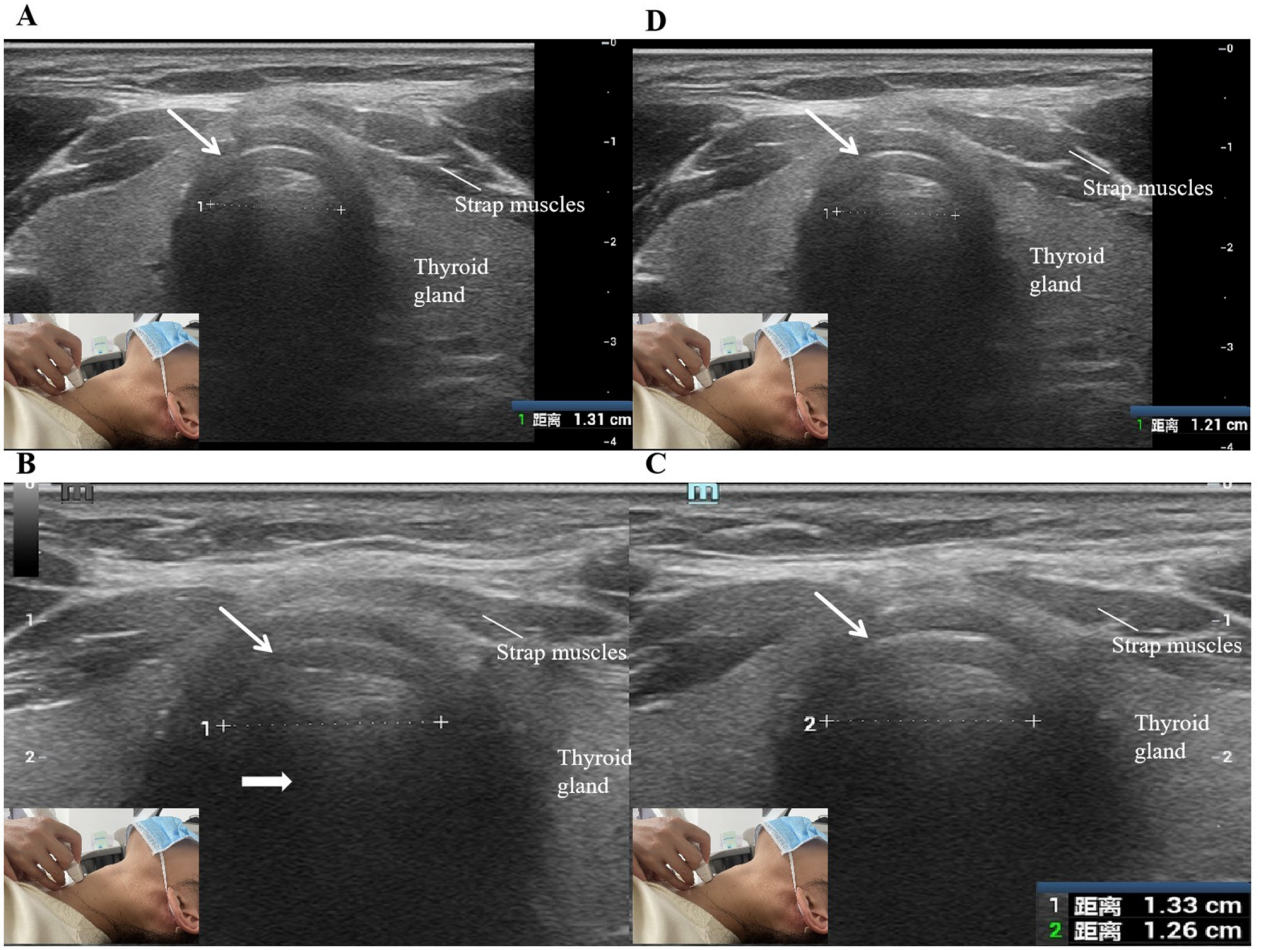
(A) Transverse sonograms of the cervical trachea during quiet respiration. (B) and (C) Transverse sonograms of cervical trachea during deep inspiration and expiration. (D) Transverse sonogram of the cervical trachea during Müller maneuver. Tracheal cartilage is a C-shaped hypoechoic structure (small arrows). Air within the cervical trachea produces a trail of strong echoes with characteristic air artifacts (large arrows). 1 and 2, lateral diameters of the cervical trachea (the distance from the inner edge of the right wall of the tracheal cartilage to the inner edge of the left wall). The insets show the transducer position on the skin.
